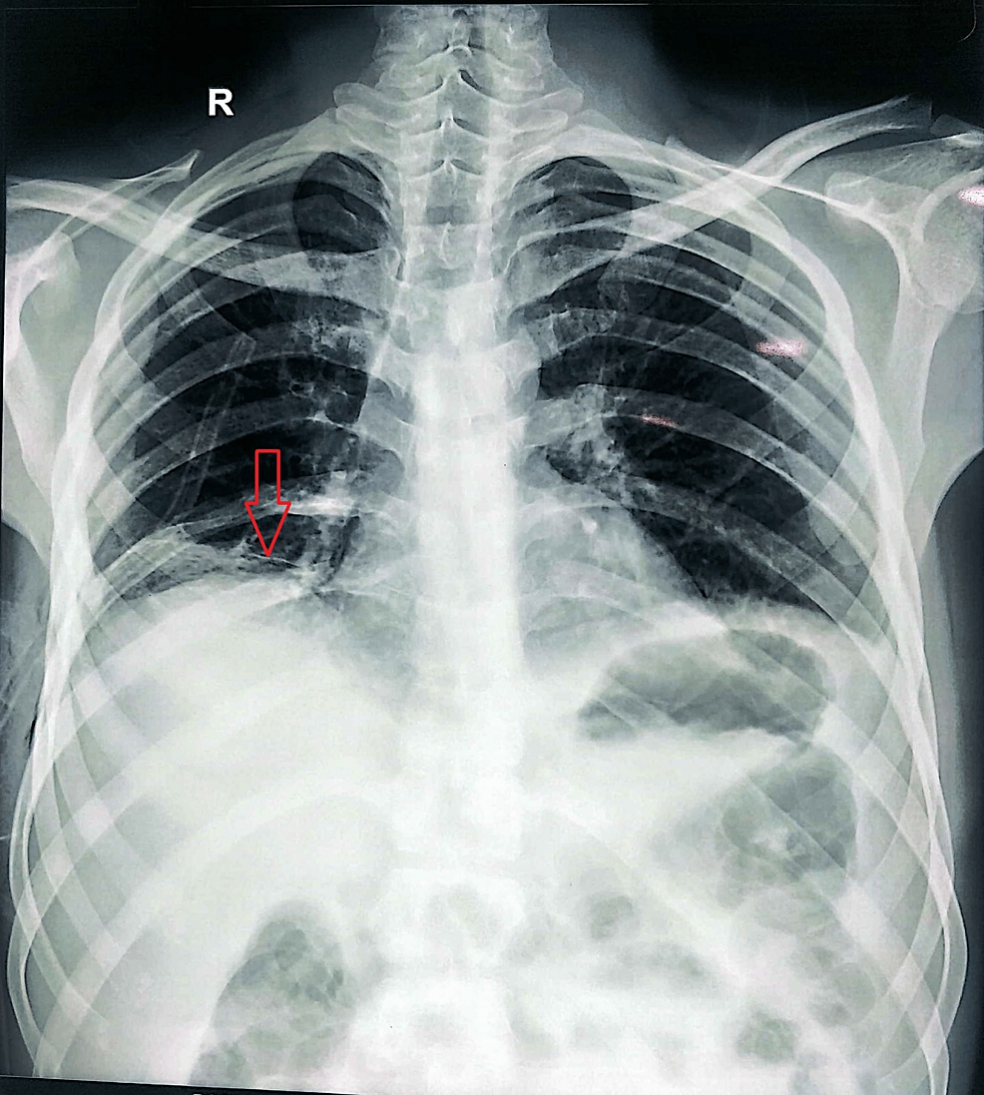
Chest X-ray post-chest-tube insertion
